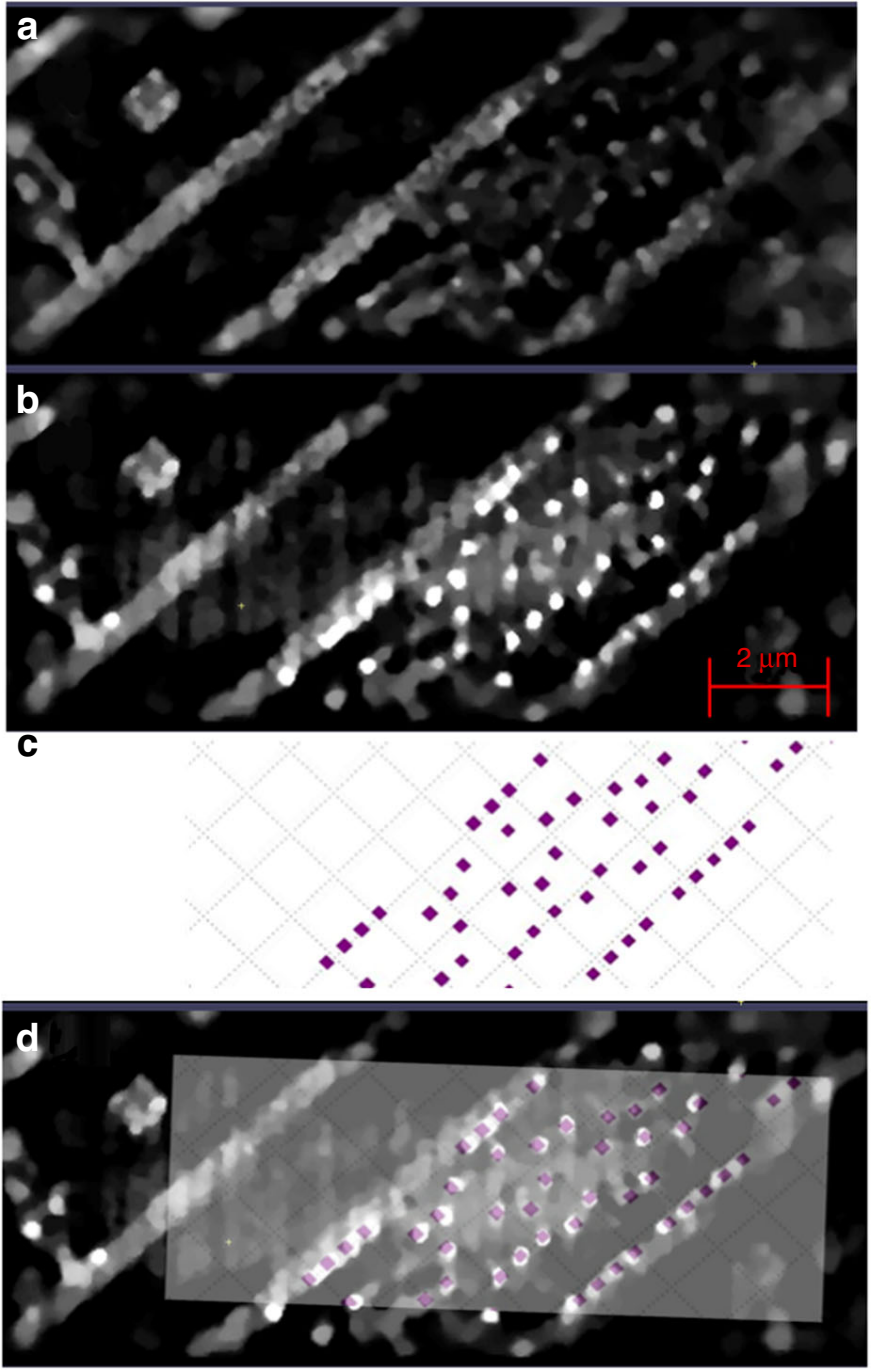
First via layer, which touches the device layer, as reconstructed (a) using 9.1 keV to 10.1 keV x-rays, (b) using 5.4 keV to 6.4 keV and 9.1 keV to 10.1 keV x-rays, (c) expected vias from GDS layer, and (d) expected vias from panel (c) shown overlaid on reconstructed layer of panel (b). The scale bar is 2 *μ*m
